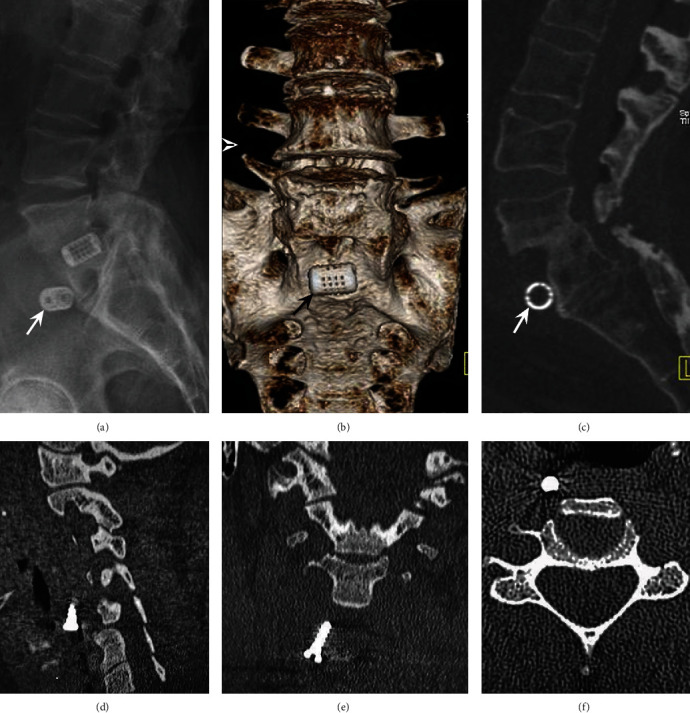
Examples of migration of spinal hardware at the lumbar and cervical spine. (a) X-ray, lateral view of the lumbar spine. (b) 3D CT reconstruction of the sacrum frontal view. (c) Multiplanar CT reconstruction, sagittal view using the bone window. (d) Multiplanar CT, sagittal view showing anterior displacement of a cervical screw at the level of C5-C6. (e) Multiplanar CT, anterior oblique view shows the right displacement of a screw outside the cervical spine. (f) Multiplanar CT, axial view showing anterior and lateral displacement of a cervical screw.
